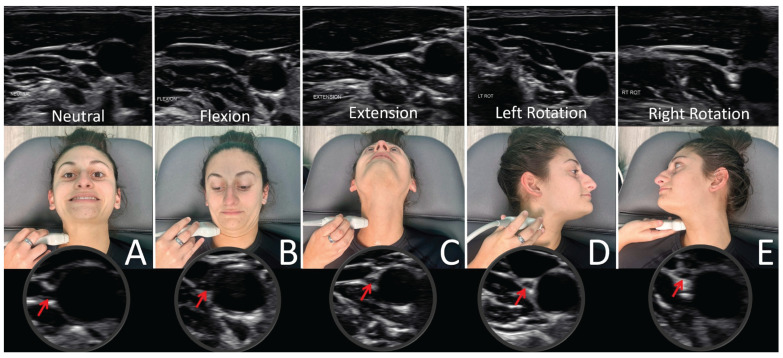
Ultrasound of vagus nerve (red arrows) in mid-cervical region with various neck positions. (A) Neck in neutral position. (B) Flexed neck. (C) Neck extended. (D) Neck rotated left. (E) Neck rotated right. As can be seen, the vagus nerve within the carotid sheath undergoes various structural tensions depending on neck positions. Figure copyright belongs to authors. (Reproduced from Ref. [29]).
